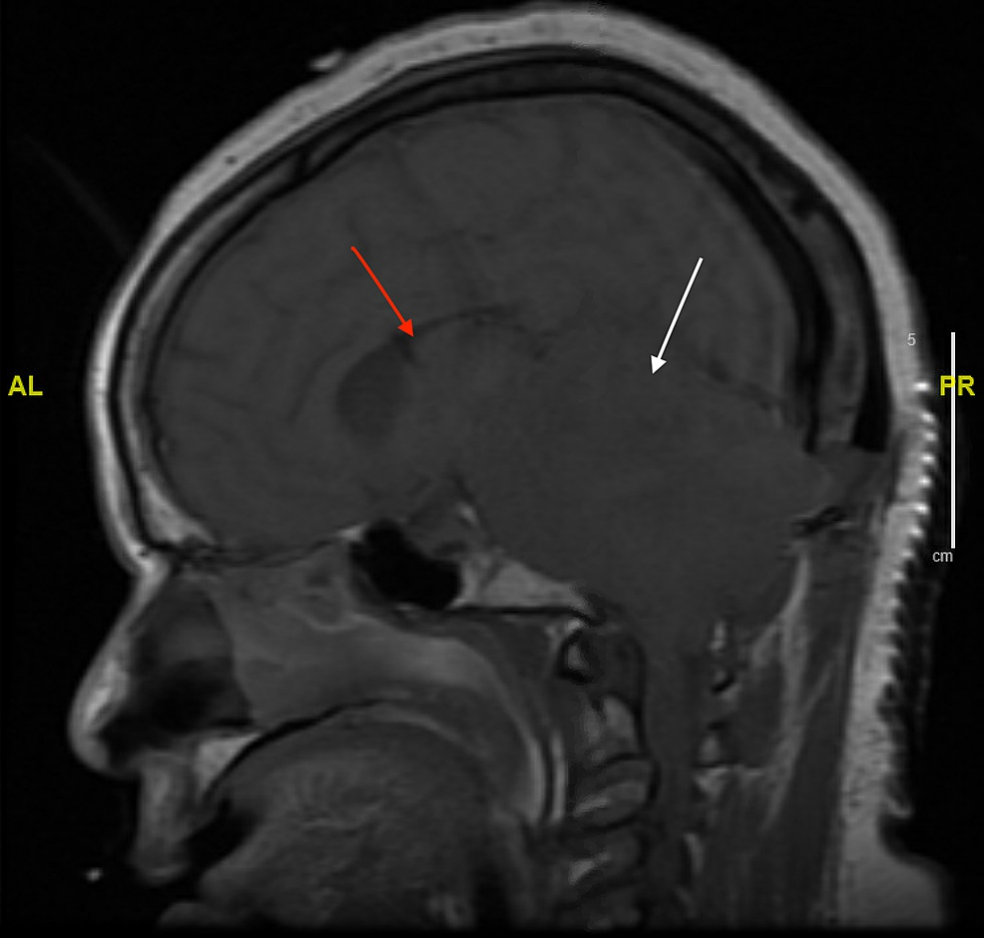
MRI of the brain without contrast in sagittal view three days into hospitalization The image shows upward and downward herniation of the cerebellar tonsils (white arrow) and mass effect on the ventricles (red arrow).
